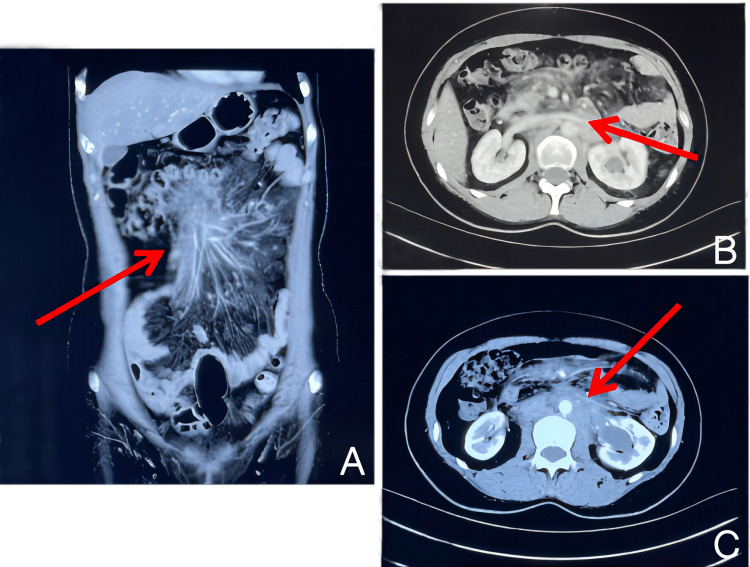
Abdominal CT of March, 2017. Soft tissue in the retroperitoneum, which invaded the small intestine (A) and, surrounding the bilateral ureters, caused bilateral hydronephrosis (B,C).
